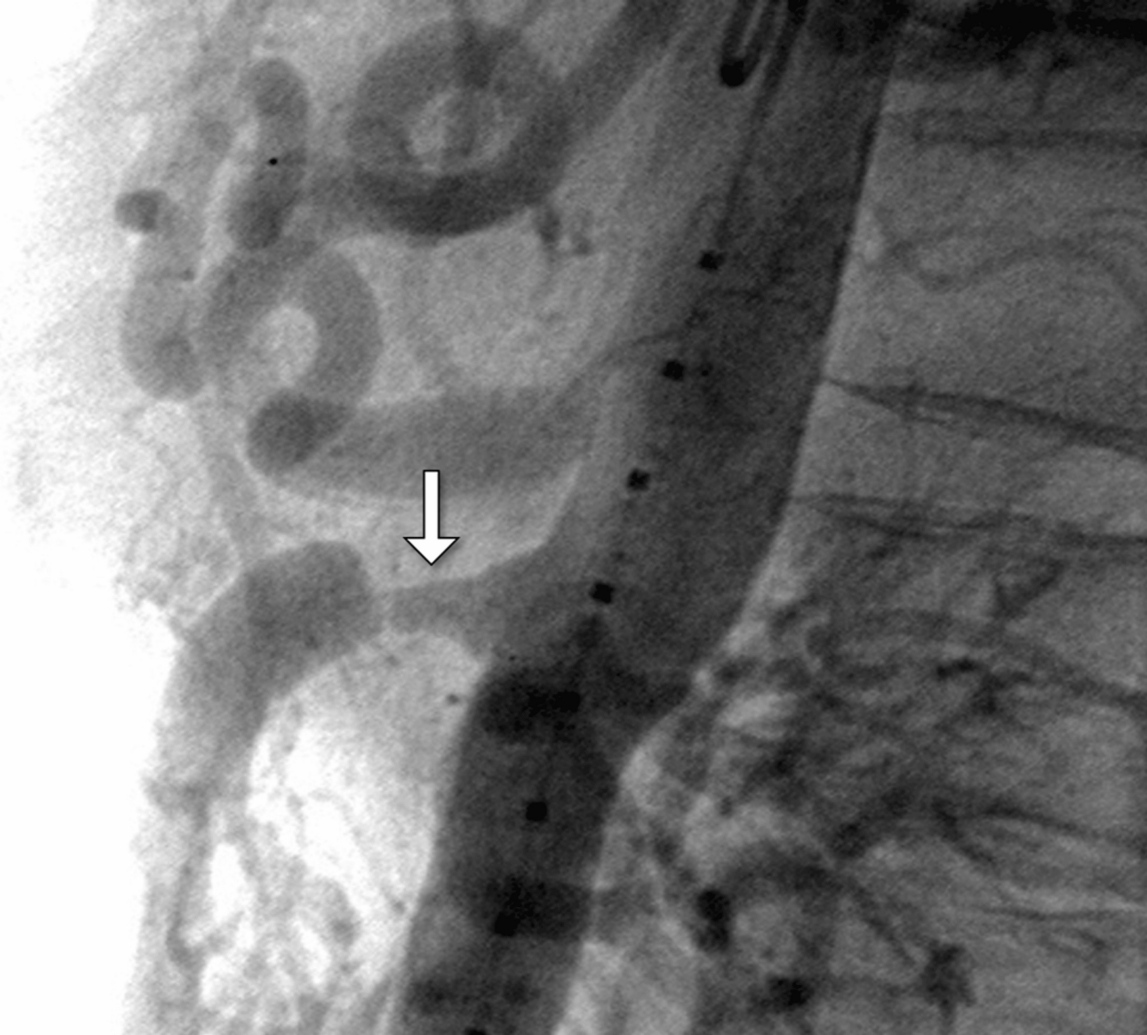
DSA confirming SMA stenosis. DSA of the mesenteric vessels reveals severe focal narrowing of the SMA (arrow) with preserved distal flow. The findings correlate with the CT evidence of mesenteric ischemia. DSA: digital subtraction angiography; SMA: superior mesenteric artery.
